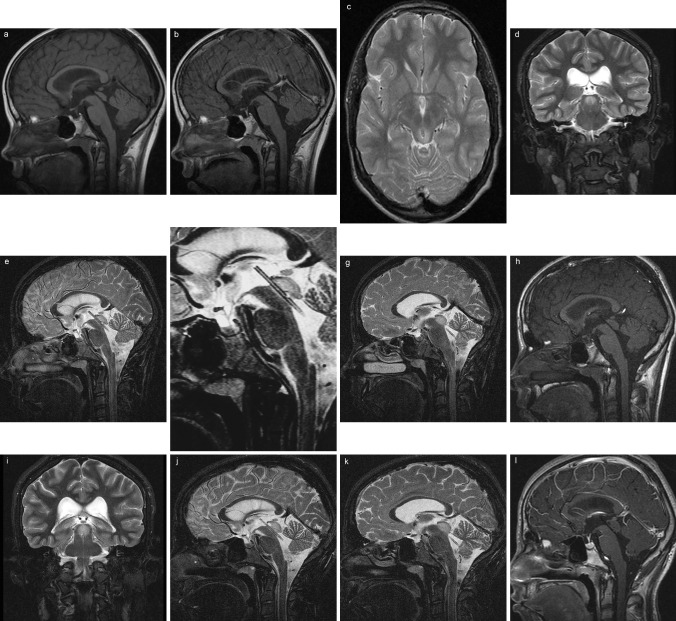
Postoperative MR images obtained 2 years a, b, 6 years c, d, e, f, g, h, and 9 years i, j, k, l after transaqueductal stenting, respectively, showing consistently the optimal position of the stent and its functionality
